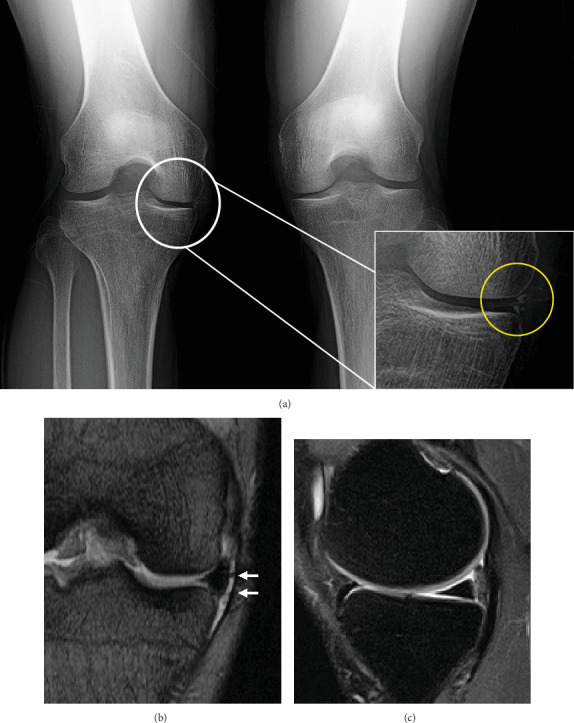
A 43-year-old male who underwent meniscal repair 28 years ago for a bucket handle tear of the MM in the right knee. (a) Preoperative plain radiograph using the Rosenberg view shows no significant osteoarthritic changes except for calcification of the medial joint space (yellow dotted circle). (b) MRI reveals infiltration of joint fluid at the interface between the MM and the joint capsule without cartilage damage. (c) In the sagittal view, no apparent tearing or degenerative changes in the posterior horn of the MM are observed. MM, medial meniscus.
